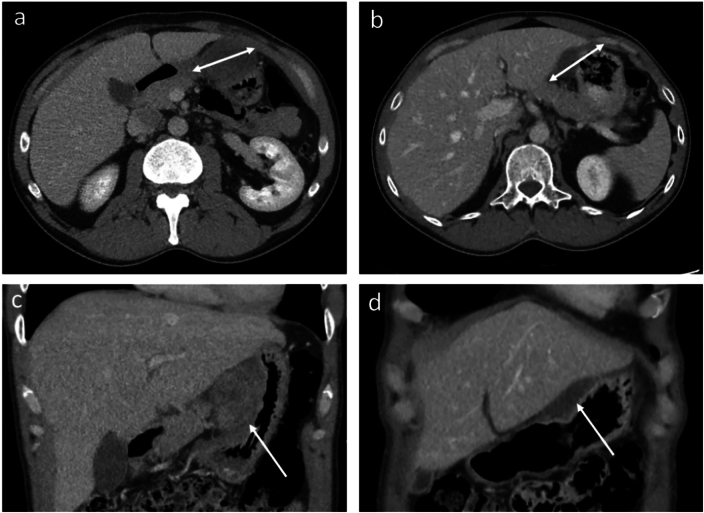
a-b) Axial portal venous phase CE-CT images of a patient diagnosed with a low-risk gastric GIST. a) Baseline CE-CT image where the transaxial tumour diameter measured 59 mm. b) Preoperative CE-CT image after 6.7 months of TKI treatment. The transaxial tumour diameter equalled 62 mm, which would suggest tumour growth rather than tumour shrinkage. c-d) However, when looking at the coronal portal venous CE-CT images, a significant decrease in tumour volume (arrows) can be observed between the c) baseline CE-CT image and d) final preoperative CE-CT image. The percentage change in tumour volume equalled 82 %.
